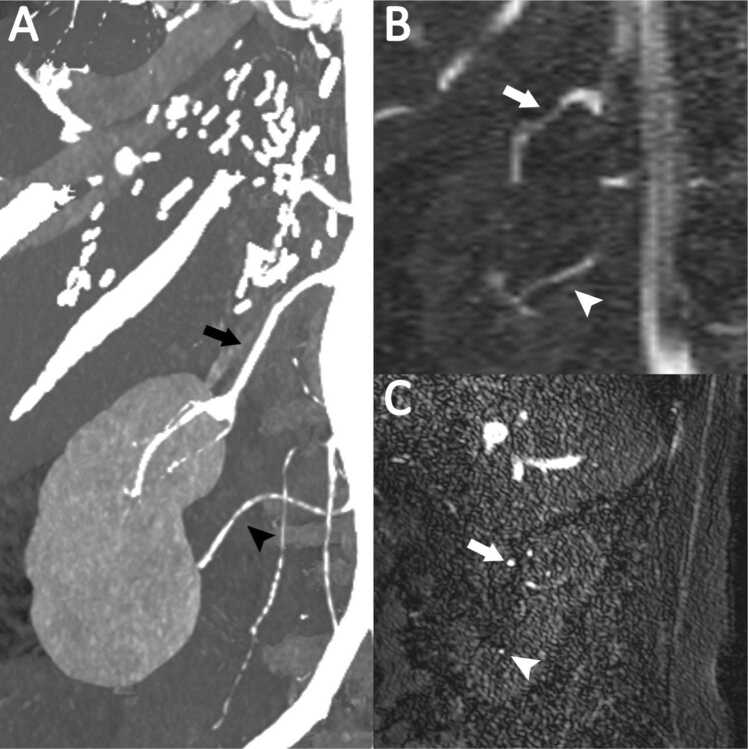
72 year-old female with aberrant right renal artery. (A) MIP Reconstruction of Photon-Counting CTA demonstrating main renal artery supplying the superior pole (arrow) and aberrant renal artery supplying the inferior pole (arrowhead). Main renal artery (arrow) and aberrant renal artery (arrowhead) are also visible in the QISS MRA coronal MIP (B) and the corresponding QISS MRA sagittal slices (C).
